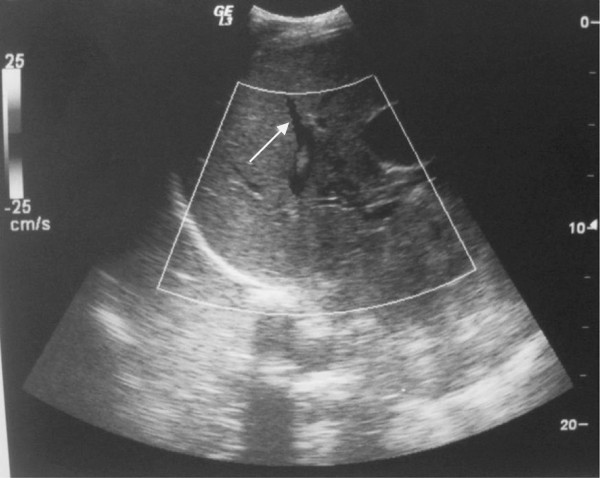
Abdominal ultrasound revealed a dilatation of the biliary ducts.
